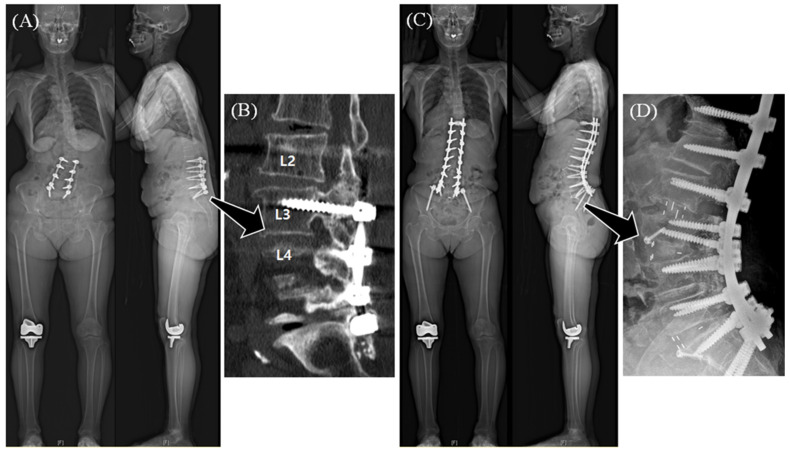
(A) A case of a 79-year-old female with iatrogenic flatback deformity. (B) A solid posterior fusion mass across L2 to L5 was seen in the preoperative computed tomography scan. (C) Revisional deformity correction was performed through a posterior–anterior–posterior approach. (D) Fusion mass osteotomy was performed, followed by lateral lumbar interbody fusion and anterior column realignment for the L2-3 and L3-4 levels, respectively. No additional fusion procedure was performed for the L4-5 level. Therefore, only the L2-3 and L3-4 levels were included in the analysis, while the L4-5 segment was not.
